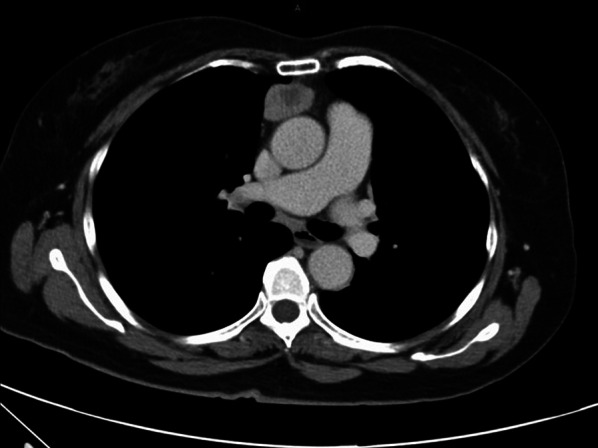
Chest computed tomography scan just before the operation revealing that the mass had regressed in size in the past month
